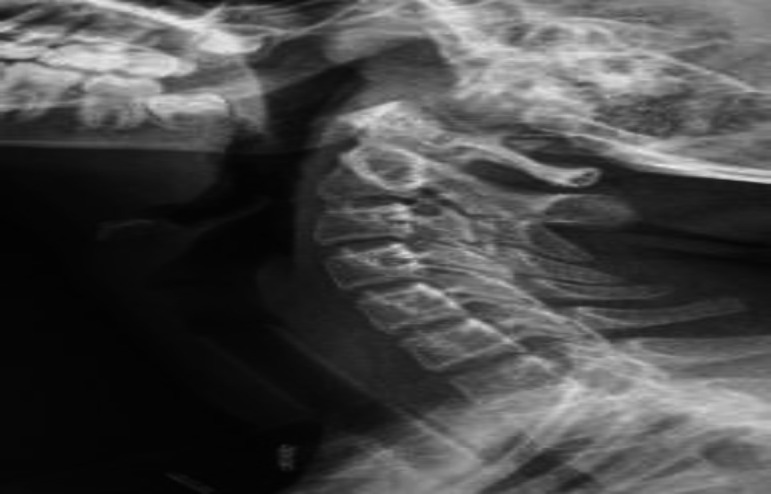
Soft tissue neck X-Ray showing Montgomery T-tube placed as a stent following Coblation release of stenosis
